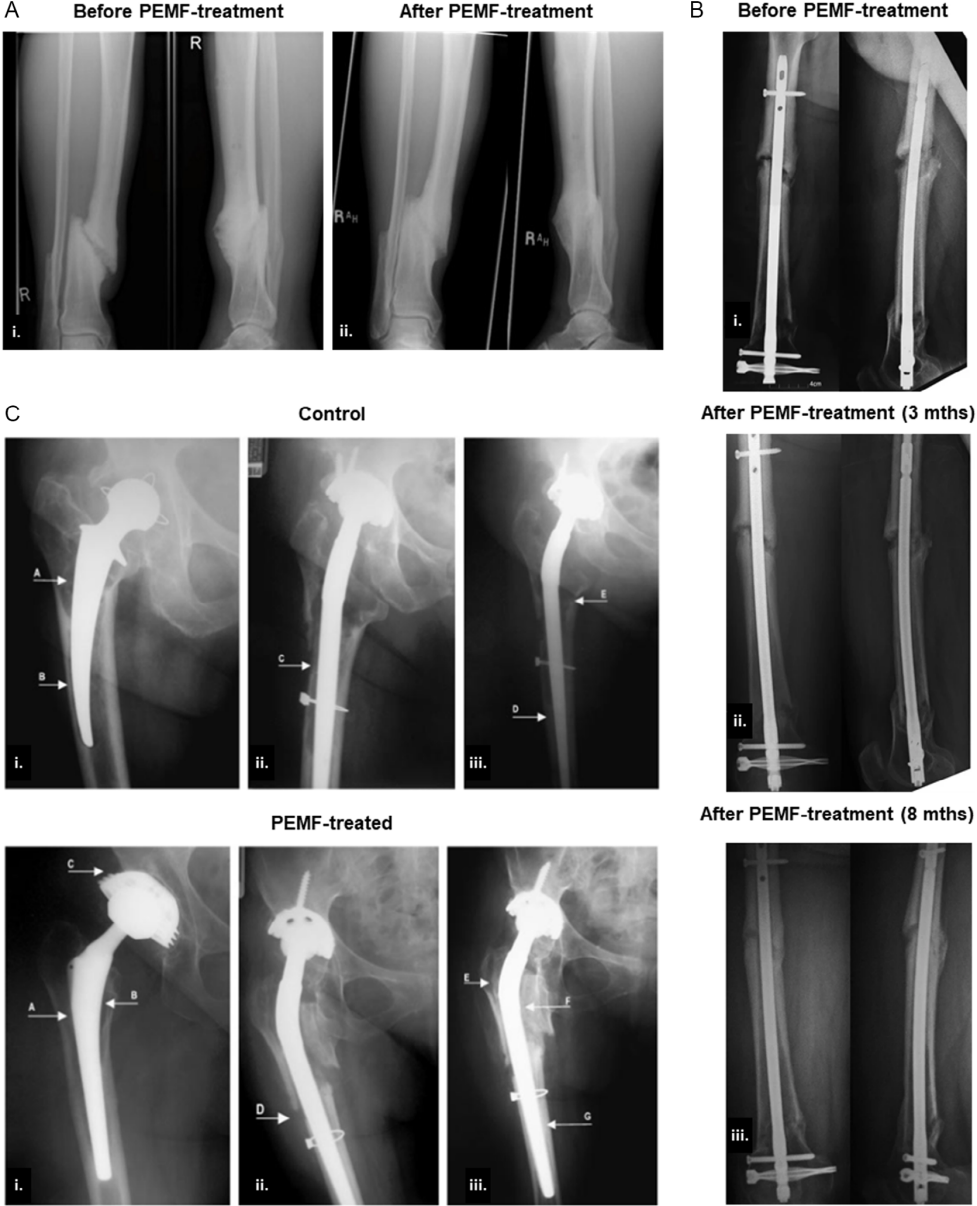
Examples of biological outcomes assessed in IC stimulation studies in humans. A) Anteroposterior and lateral radiographs of a distal tibial nonunion: i) 10 months after fracture; ii) after PEMF stimulation of the fracture site for 5 months, leading to fracture union. Reproduced and adapted with permission.^[^
